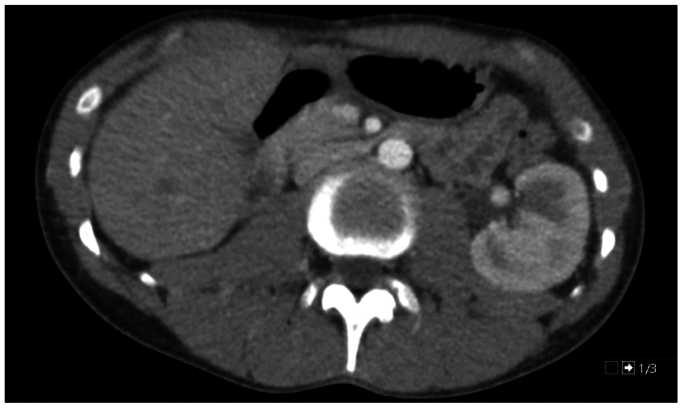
Abdominal CTA revealing tapering of the left renal vein (LRV).
